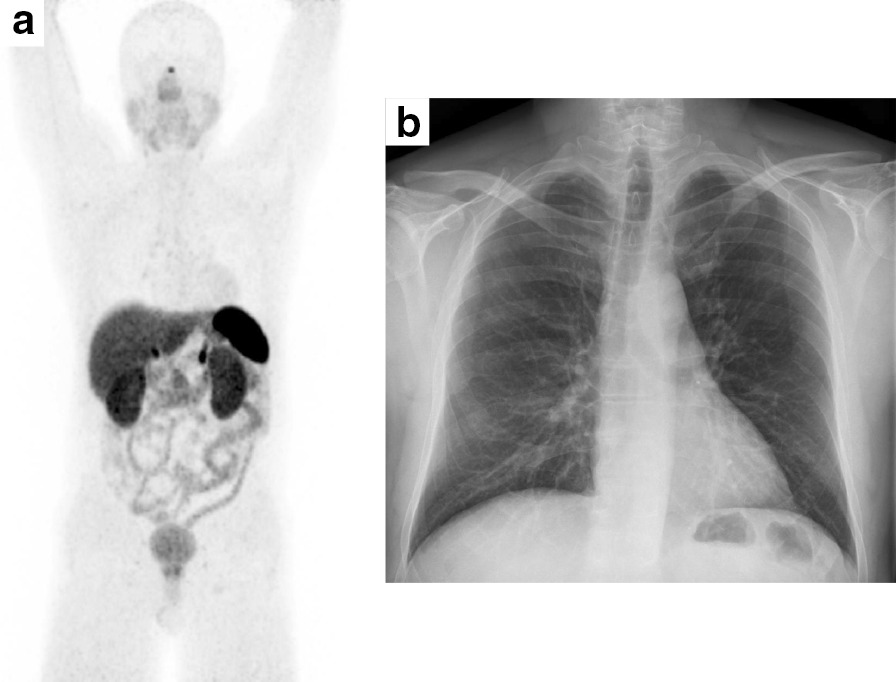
Imaging studies in the context of aftercare. Imaging studies show no signs of primary testicular carcinoid tumor recurrence 3 years DOTA-TATE-PET/CT (a) and 10 years Chest X-ray (b) after initial diagnosis
